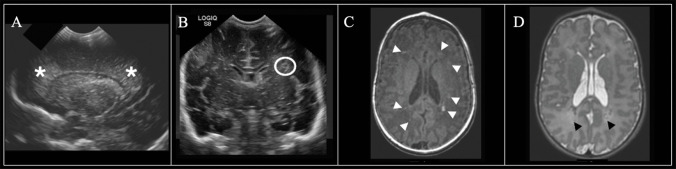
Sequential neuroimaging in a female infant born at 30 weeks. (A) 7-DOL coronal-plane head ultrasound demonstrated diffusely increased white matter echogenicity equal to or brighter than choroid plexus (*asterisk*, Miller score 4); (B) 30-DOL coronal-plane head ultrasound showed cavitary cystic change in the white matter (*white circles*, Miller score 5); term-equivalent age MRI demonstrated (C) greater than 6 foci of increased signal intensity in the white matter on axial T1-weighted imaging (*white arrowheads*) and (D) small periventricular cysts (*black arrow*) on axial T2-weighted imaging with decreased white matter volume (Martinez-Biarge score 2)
